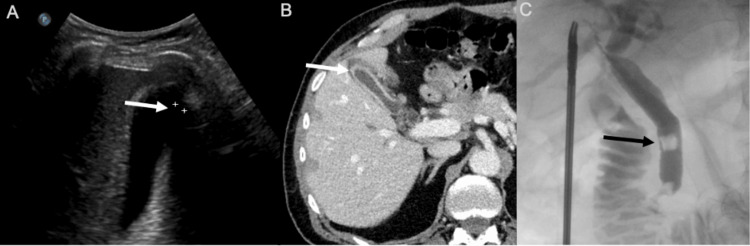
(A) Ultrasound examination of the RUQ revealed mild thickening of the gallbladder wall without cholelithiasis and dilation of the CBD to 9.3 mm without choledocholithiasis. The full length of the CBD could not be visualized using ultrasonography. (B) Contrast-enhanced CT scan of the abdomen showed gallbladder wall thickening and possible sludge or non-calcified stones in the distal portion of the CBD. (C) IOC revealed multiple gallstones in the CBD RUQ: right upper quadrant; CBD: common bile duct; IOC: intraoperative cholangiogram
